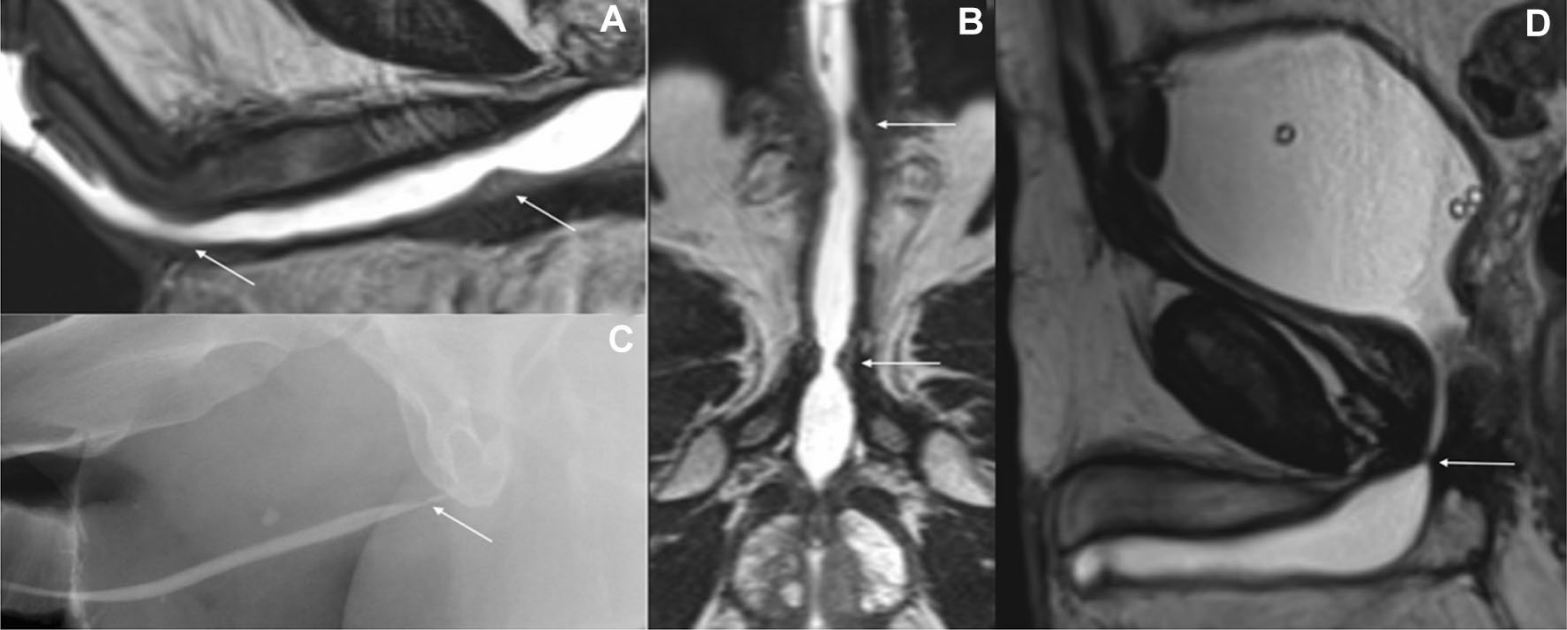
MRU sagittal T2-WI image (A) and reformat through the lumen of the urethra (B) present two stenoses within the bulbar part and at the border of the bulbar and penile part with surrounding fibrosis (arrows). C VCUG. Stenosis in the bulbar part of the urethra is also visualized in urethrography. D MRU sagittal T2-weighted image presents a short stenosis (arrow) in the bulbar part of the urethra, impossible to demonstrate in SUG
